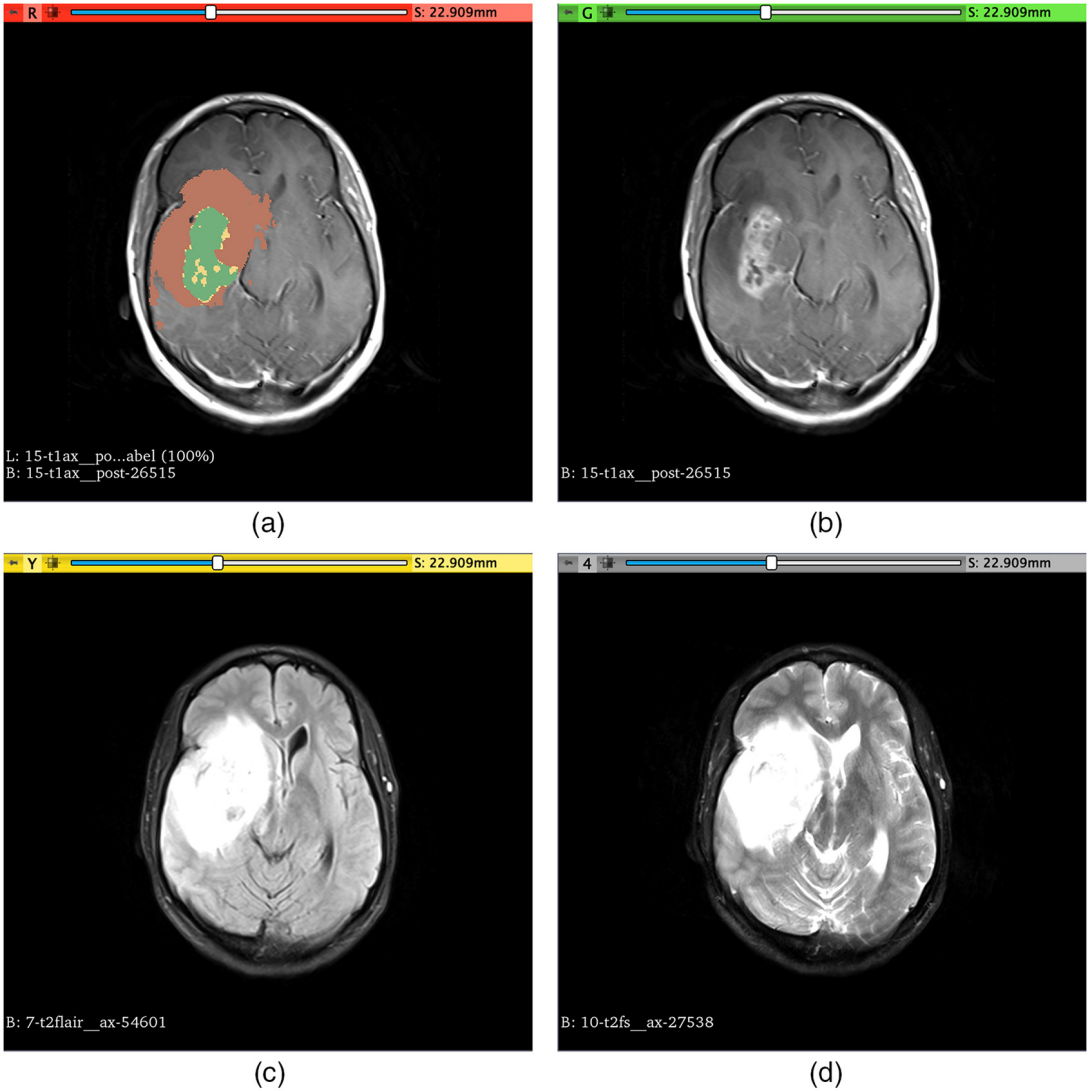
Example of segmentation of the different regions used to extract the radiomic features (TCIA case ID – TCGA-14-3477) overlaid in the cT1w image (a). Enhanced tumor region (L1) is shown in green, nonenhanced tumor and necrosis (L2) are shown in yellow, and edema (L3) is shown in brown. (b) The cT1w image without segmentation overlay; (c) FLAIR image; (d) T2w image.
